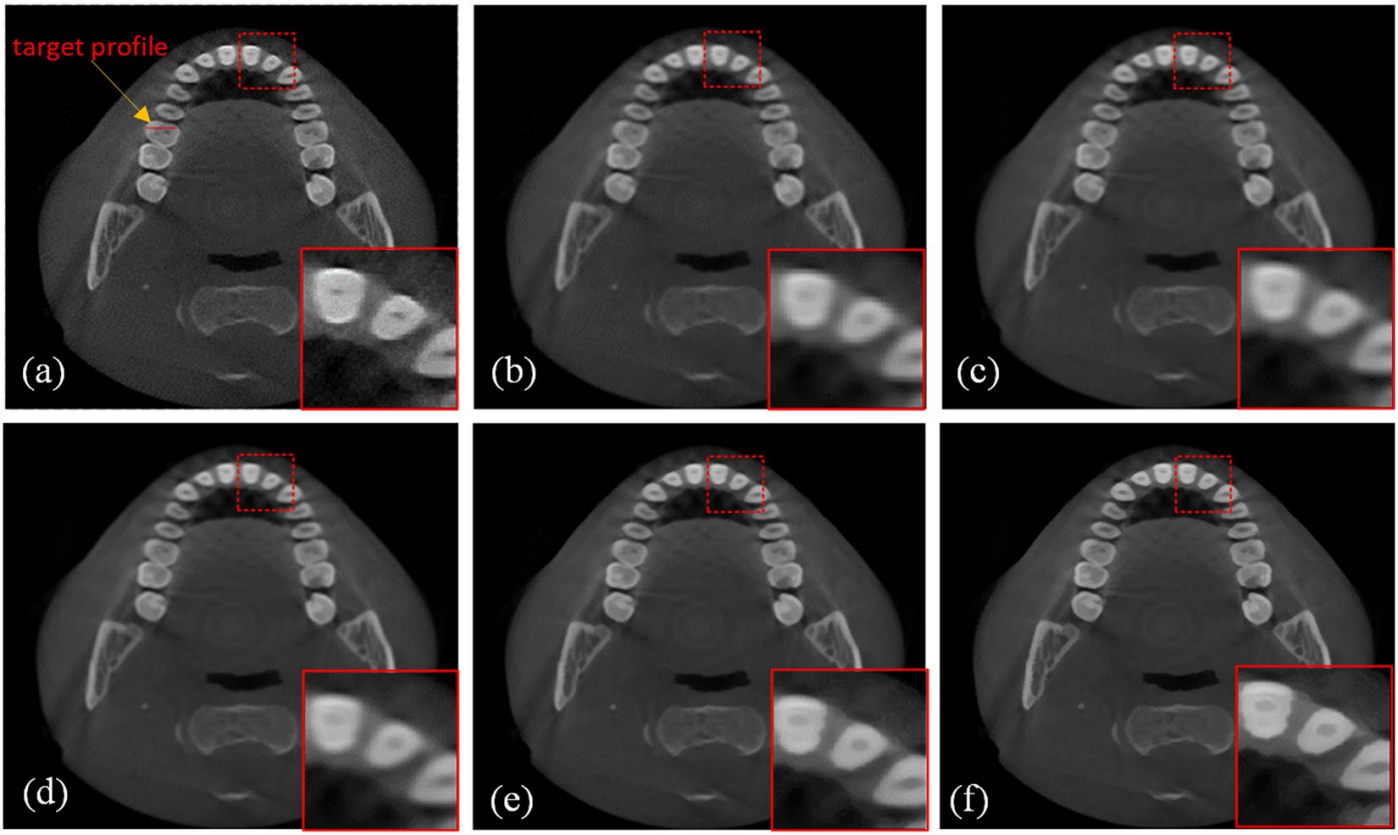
Comparison of SR reconstructed images of dental CT images. (a) Original CT image. (b) Input LR CT image degraded from (a). (c) Reconstructed image obtained using the bicubic interpolation method (PSNR = 35.0198, RMSE = 4.5243, SSIM = 0.8793). (d) Image reconstructed using the proposed method with 1 iteration (PSNR = 35.181, RMSE = 4.4411, SSIM = 0.8768). (e) Image reconstructed using the proposed method with 5 iterations (PSNR = 35.9691, RMSE = 4.0559, SSIM = 0.8798). (f) Image reconstructed using the proposed method with 8 iterations (PSNR = 36.1571, RMSE = 3.9691, SSIM = 0.8806). All of the images are displayed in the same window. This display window is [0, 255].
